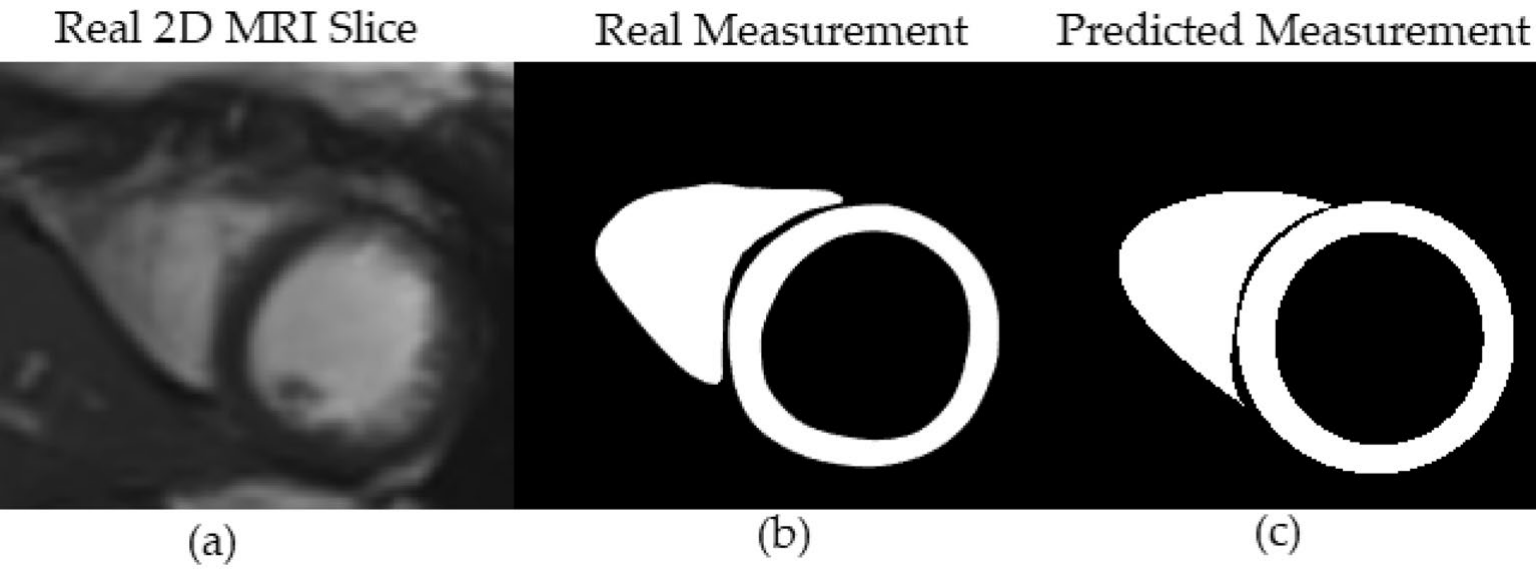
(a) The real cardiac MRI 2D image slice. (b) The segmented binary slice measurement $$z_t$$. (c) The predicted binary measurement $$\hat{z}_t$$, generated from the contours obtained via the intersection of deformable model and image slice plane.
